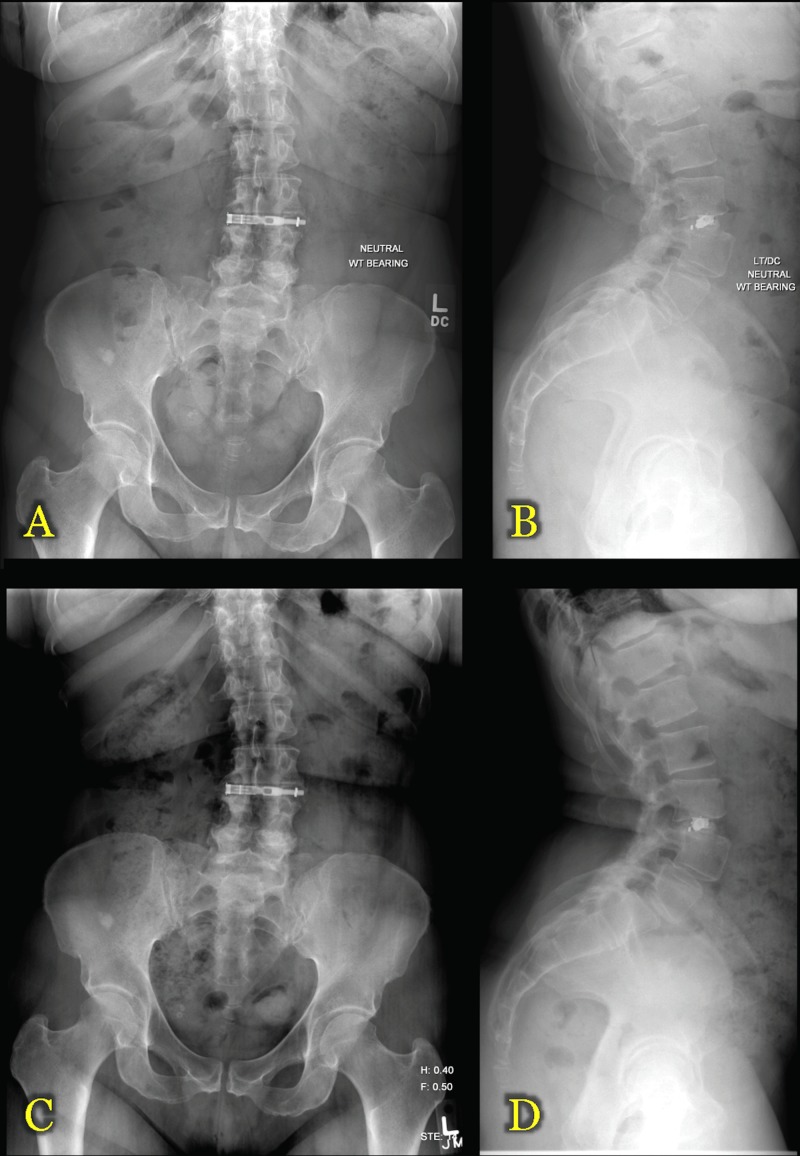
Postoperative X-Ray (XR) Imaging Panels A and B demonstrate 36" standing scoliosis anteroposterior (AP) and lateral XR images, respectively, two months postoperatively. Panels C and D demonstrate 36" standing scoliosis anteroposterior (AP) and lateral XR images, respectively, six months postoperatively.
